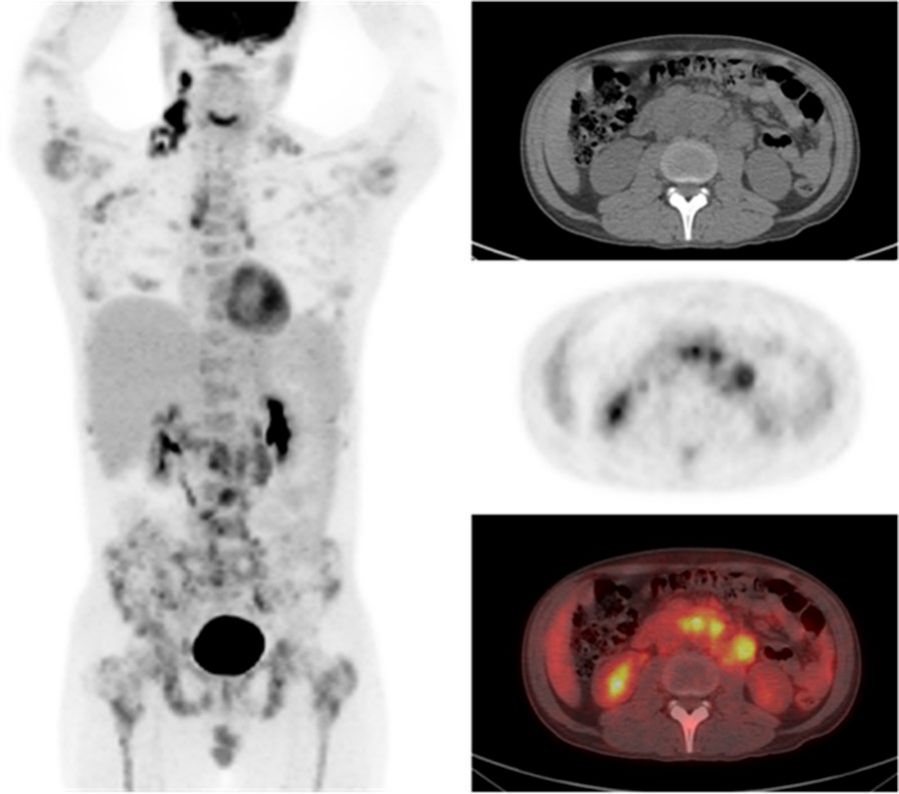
Radiology showing extent of disease burden. PET-CT showing extensive intensely FDG-avid lymphadenopathy above and below the diaphragm and extensive FDG-avid skeletal/marrow disease burden
